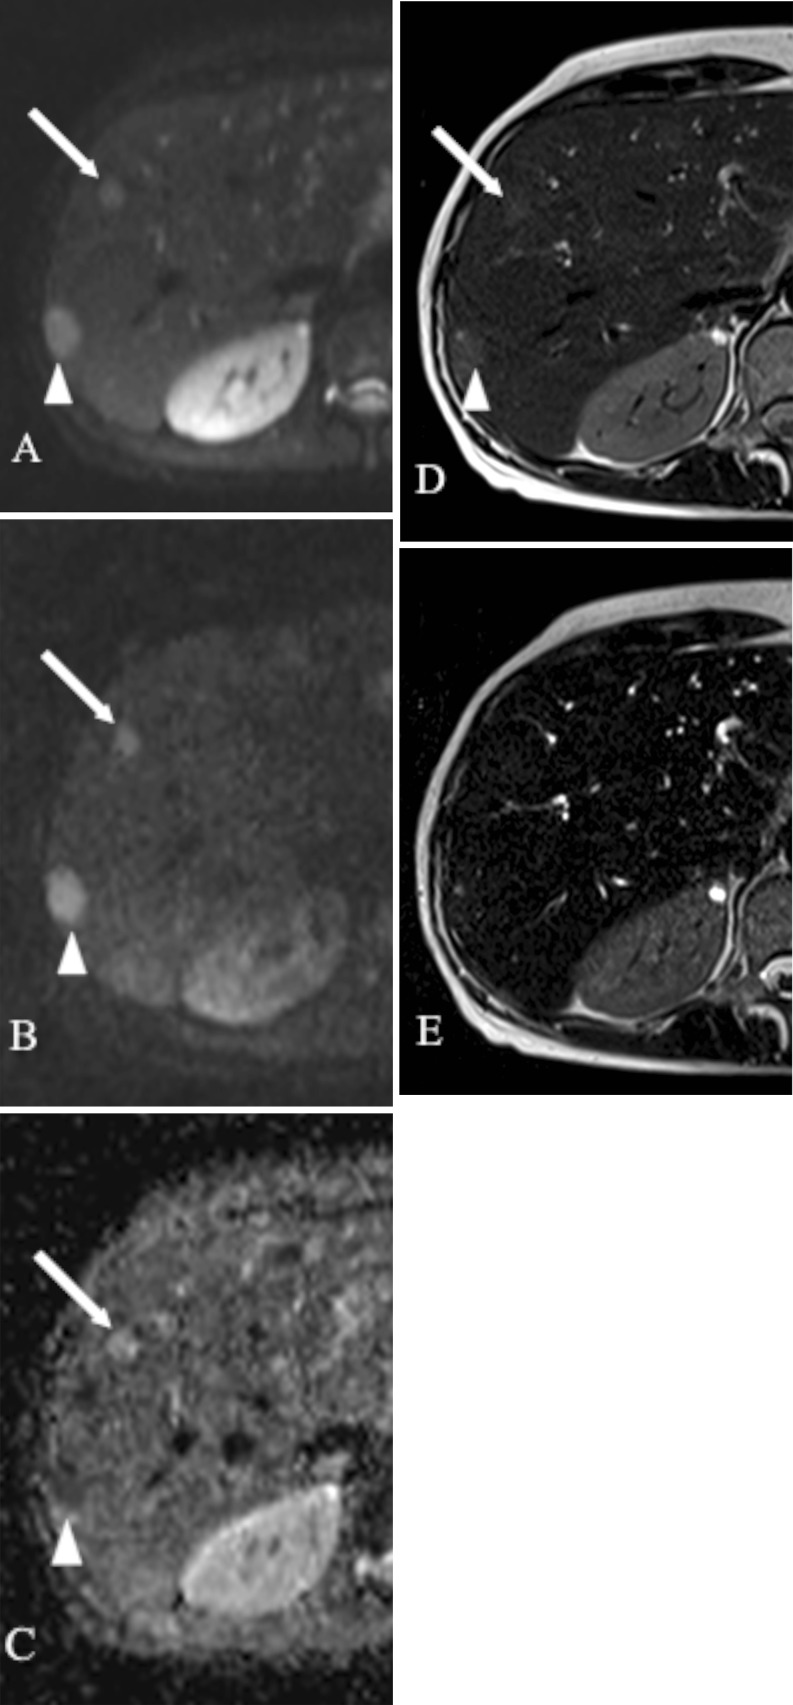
MR images obtained in a 51-year-old woman with liver metastases from neuroendocrine cancer. a
